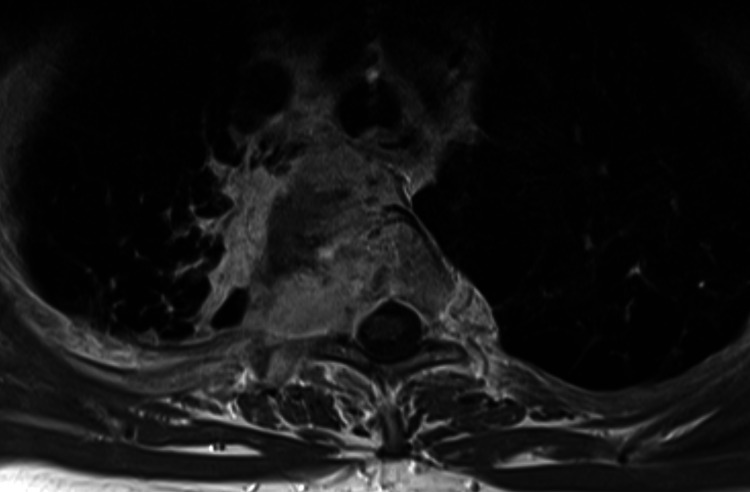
Axial contrast-enhanced T1 TSE MRI at the level of the primary lung tumor. The tumor invades the spine and the spinal canal at the right-hand aspect of the spinal cord. MRI, magnetic resonance imaging; TSE, turbo spin echo
